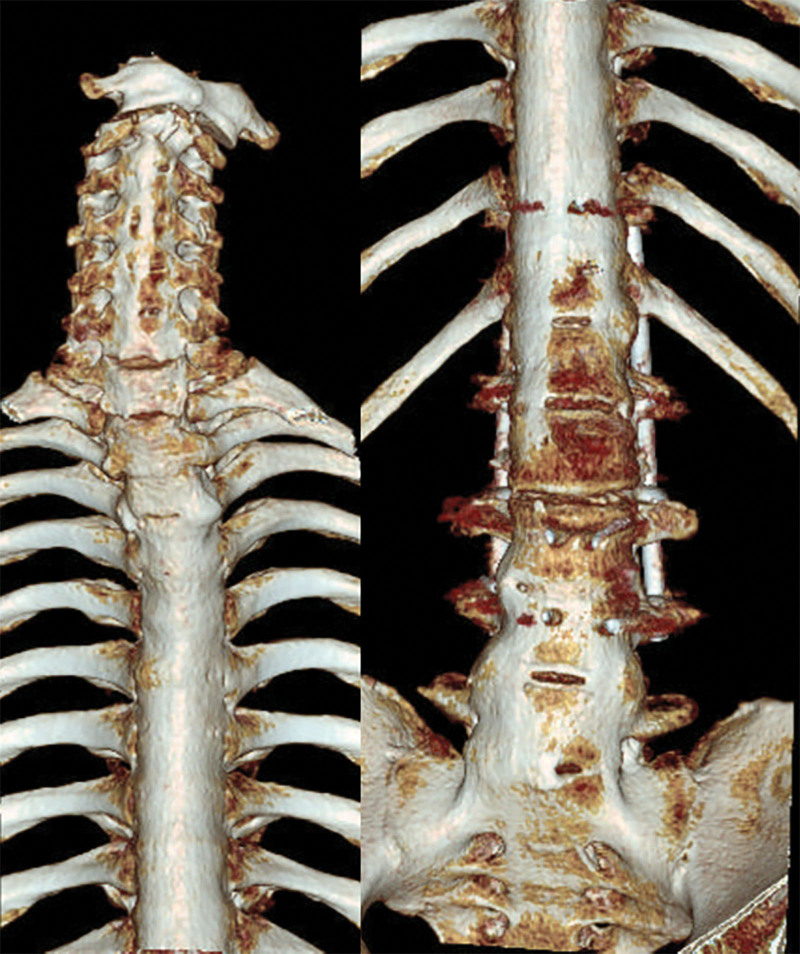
Preoperative spinal 3D computed tomography image showing ankylosis of the entire spine from the sacroiliac joint to the cervical spine.
